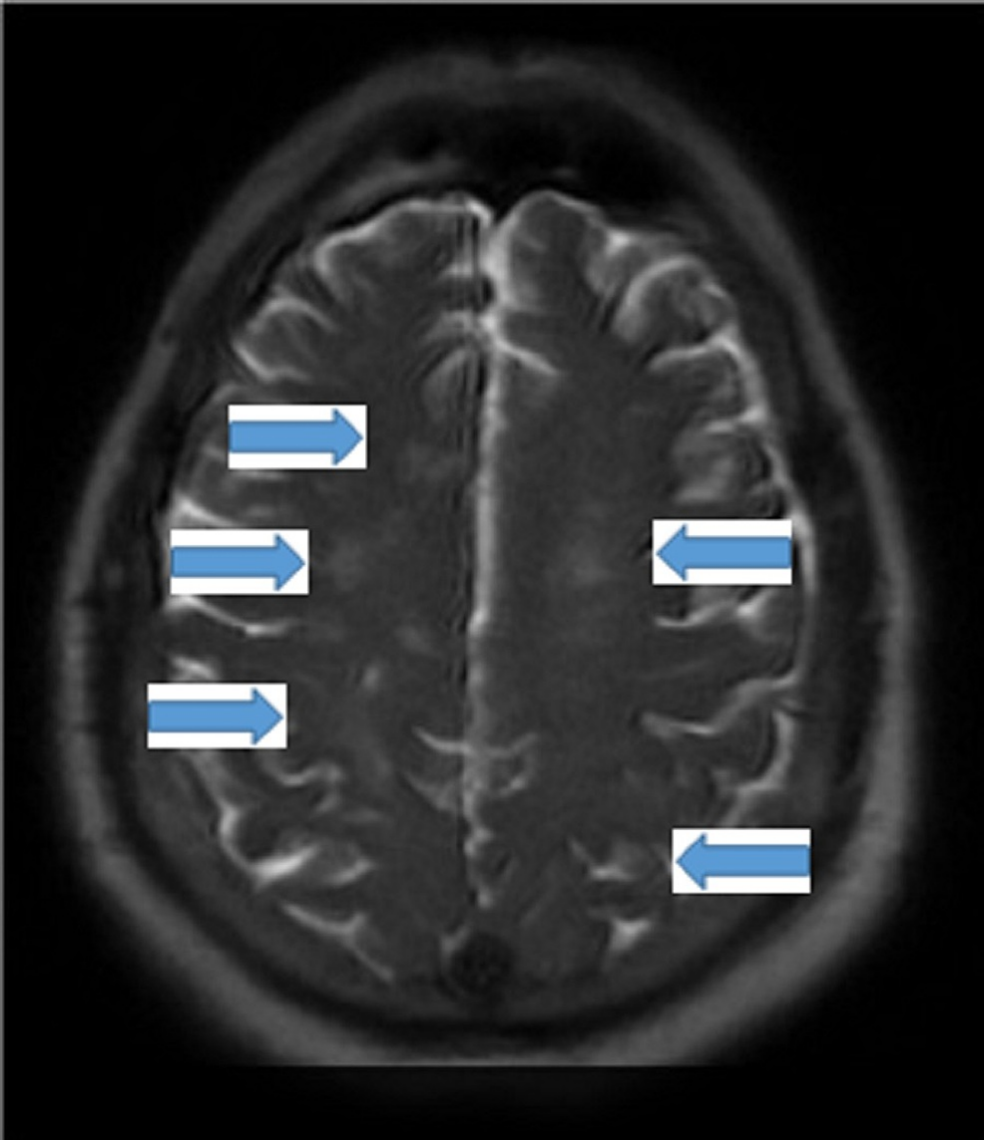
MRI brain showing multiple bilateral patchy acute infarctions (arrows) MRI: magnetic resonance imaging
